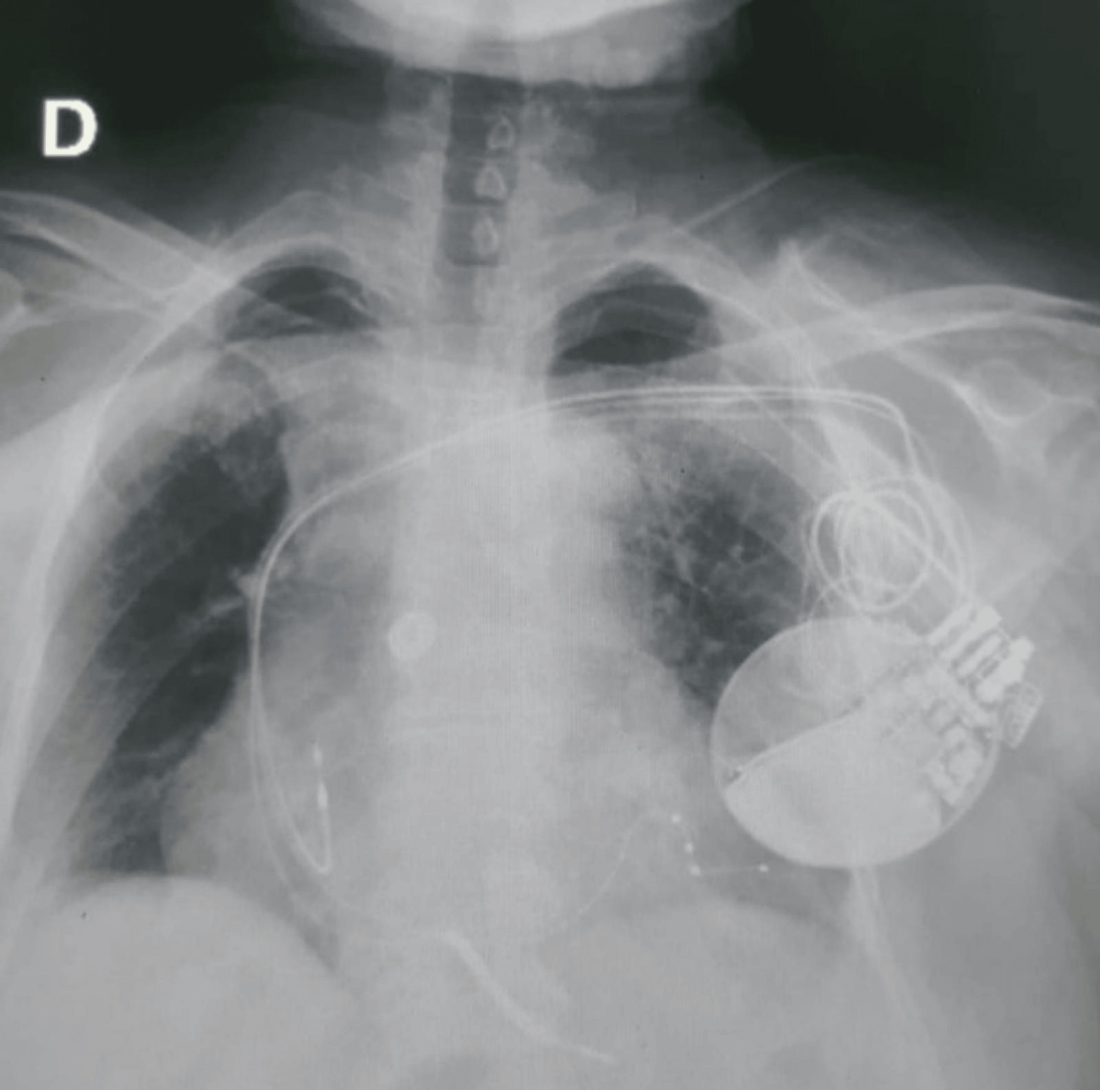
Chest radiograph showing the appropriate positioning of the cardiac resynchronization therapy defibrillator leads (RA, RV, and LV via the coronary sinus) 24 hours after implantation RA: right atrium; RV: right ventricle; LV: left ventricle
